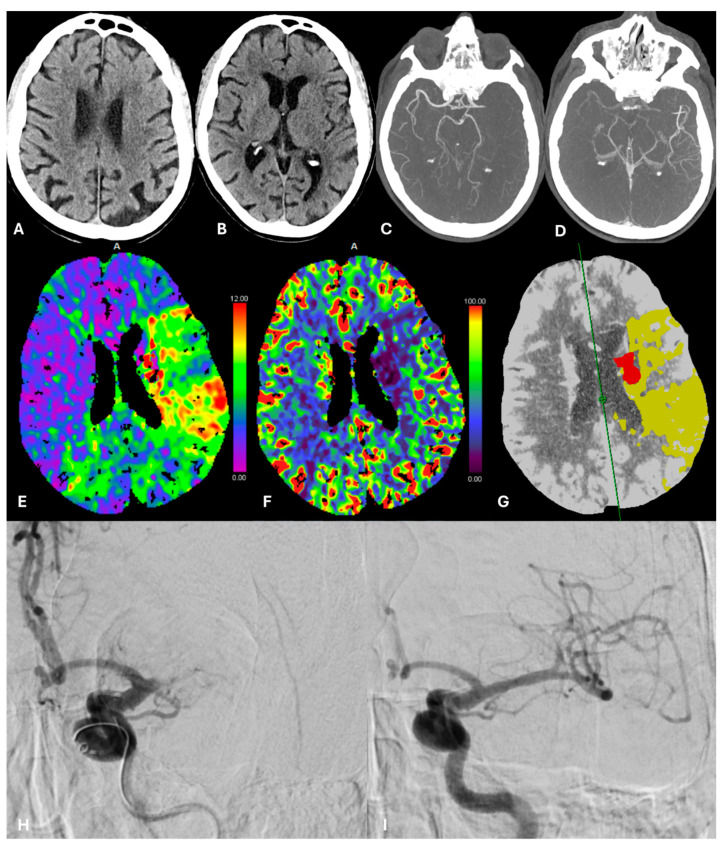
Case Nr. 10. Unenhanced CT shows a small ischemic lesion in the left lentiform nucleus, ASPECT score 9 (A,B). Multiphase angio-CT documents a left M1 occlusion (C) with good collateral circulation in the later acquisition (D), also facilitating estimates of the thrombus length. CT Perfusion: Tmax (E) and CBF (F) maps show a large hypoperfusion area in the left cerebral hemisphere; summary map (G) clearly outlines a small ischemic core (red area) and a large ischemic penumbra (yellow area), based on Tmax > 6s and rCBF < 30% respectively. Left M1 occlusion was confirmed during the diagnostic angiography (H) and treated by thromboaspiration technique, achieving complete recanalization of the middle cerebral artery (I).
